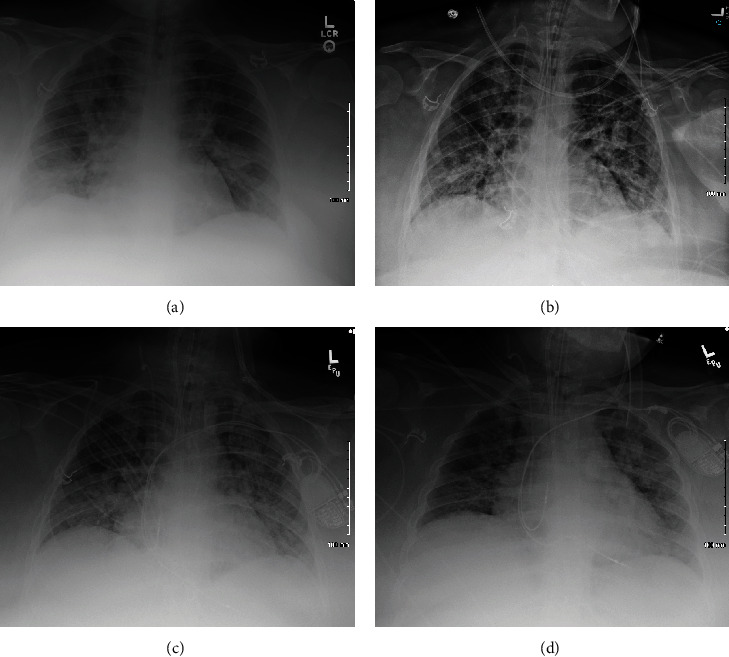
Progression of lung findings on chest radiography in postpartum patient with severe COVID 19.
